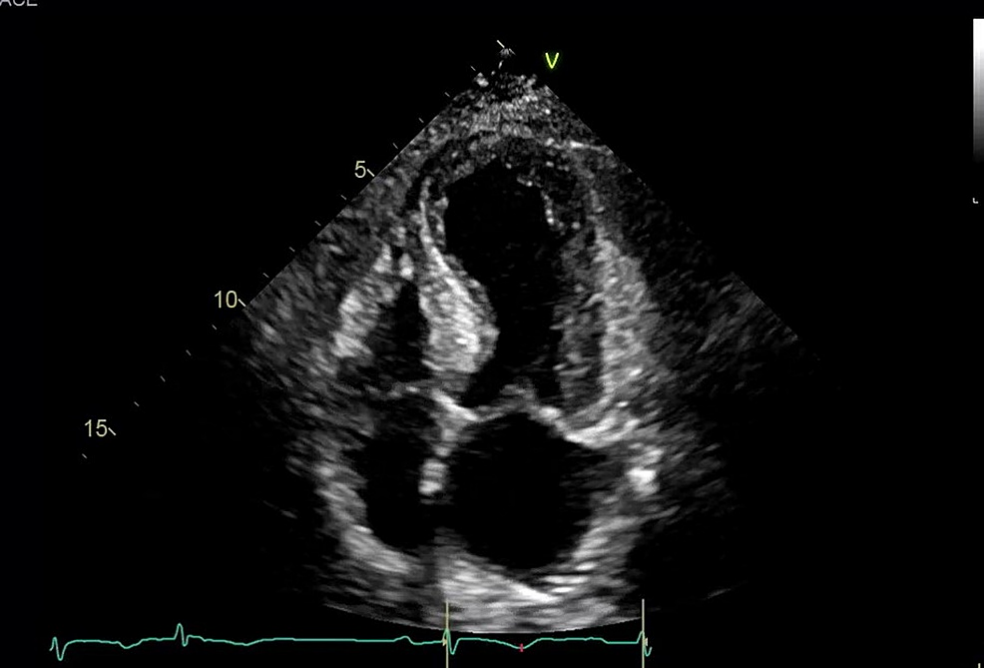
Transthoracic echocardiogram (TTE) demonstrating regional wall abnormalities of the left ventricle during systole The mid and distal anterior septum, entire apex and mid septum segment are hypokinetic. All other remaining scored segments are normal.
